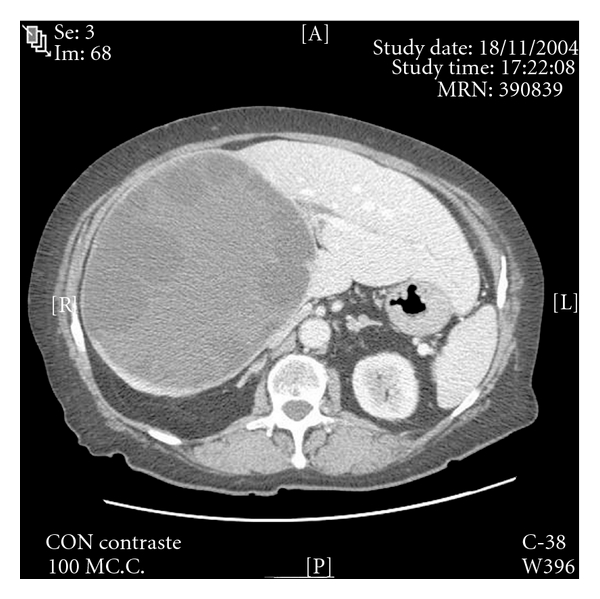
Preoperative abdominal CT. Massive liver hydatid cyst replacing segments IV to VIII.
